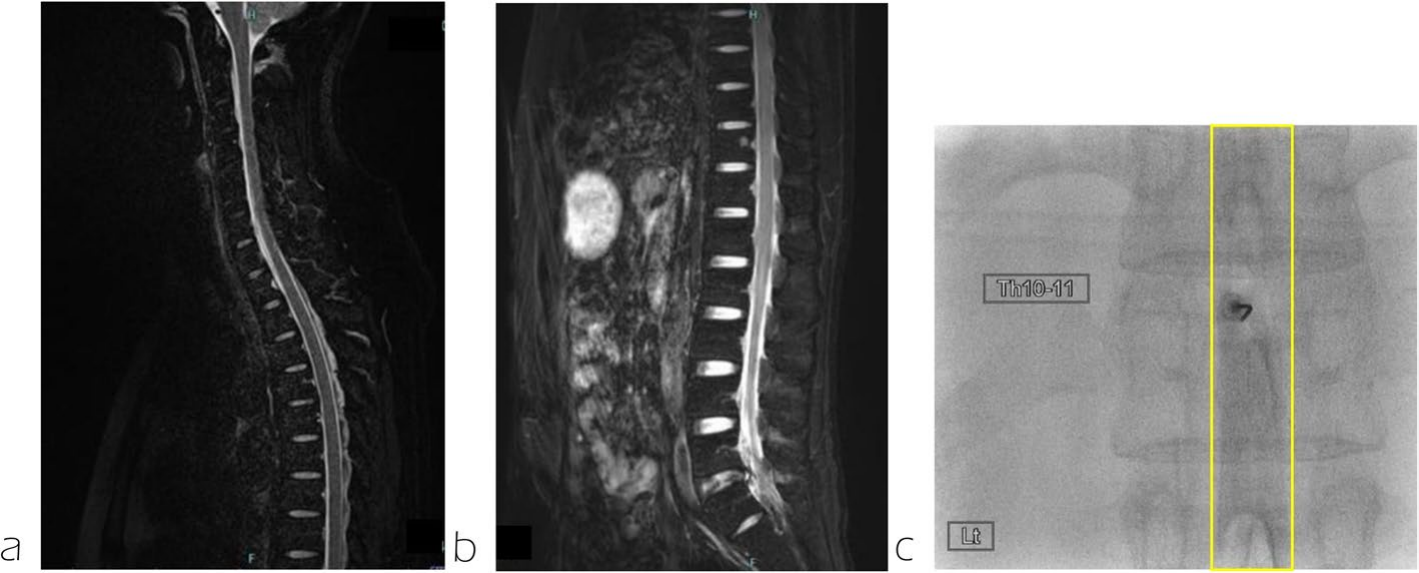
Spinal MRI, CT myelography and fluoroscopy results. a High-intensity signals were noted within the epidural space on fat-suppressed T2-weighted spinal MRI. b Epidural contrast enhancement observed on CT myelography. c Subdural contrast distribution was identified on fluoroscopy (square)
